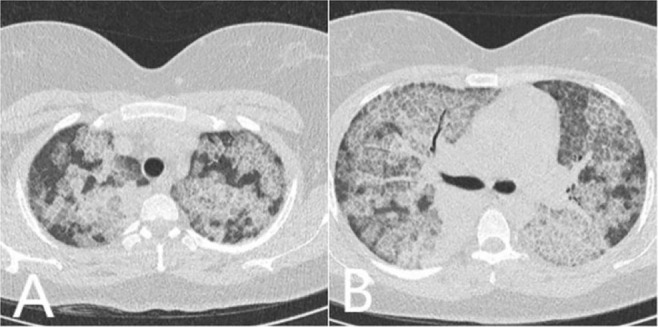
Non-contrast axial chest CT scan of a 34-year-old woman presented with progressive dyspnea and cough for three months. CT demonstrates zones of ground-glass density with interlobular septal thickening, representing typical “crazy-paving” appearance. Bronchoalveolar lavage (BAL) was positive for the accumulation of periodic acid-Schiff (PAS)-positive lipoproteinaceous material.
